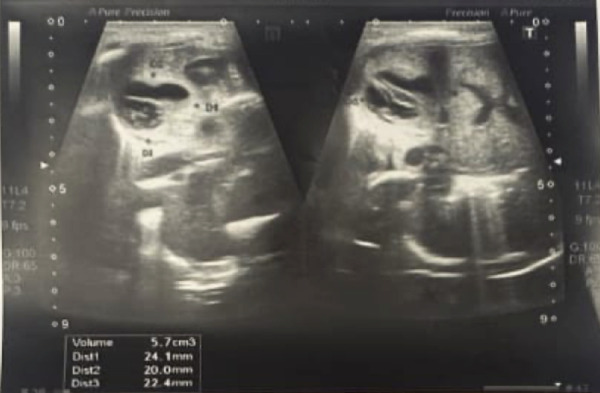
Ultrasound abdomen showing right-sided adrenal haemorrhage
